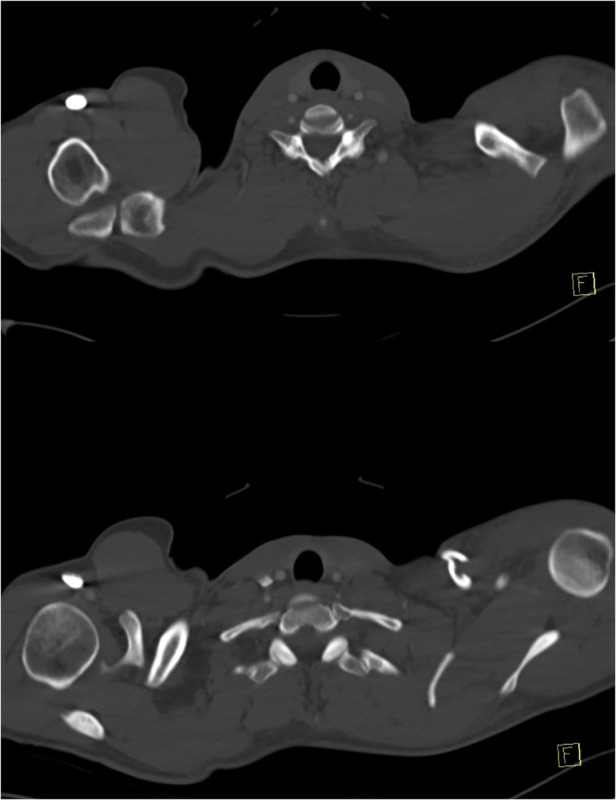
Axial computed tomography demonstrating concomitant midshaft clavicle fracture and Rockwood Type V acromioclavicular joint dislocation.
